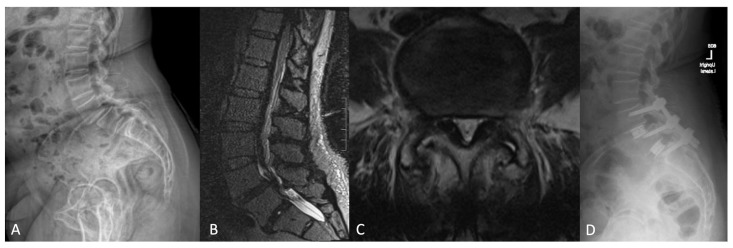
Cropped section of a preoperative lateral upright whole spine film (A) demonstrating the spondylolisthesis at L4–L5 and L5–S1. Preoperative sagittal (B) and axial (C) T2-weighted MRI slices demonstrating severe central canal and lateral recess stenosis, ligamentum flavum hypertrophy, and diffuse disc bulge. Postoperative upright lateral lumbar spine plain film (D).
